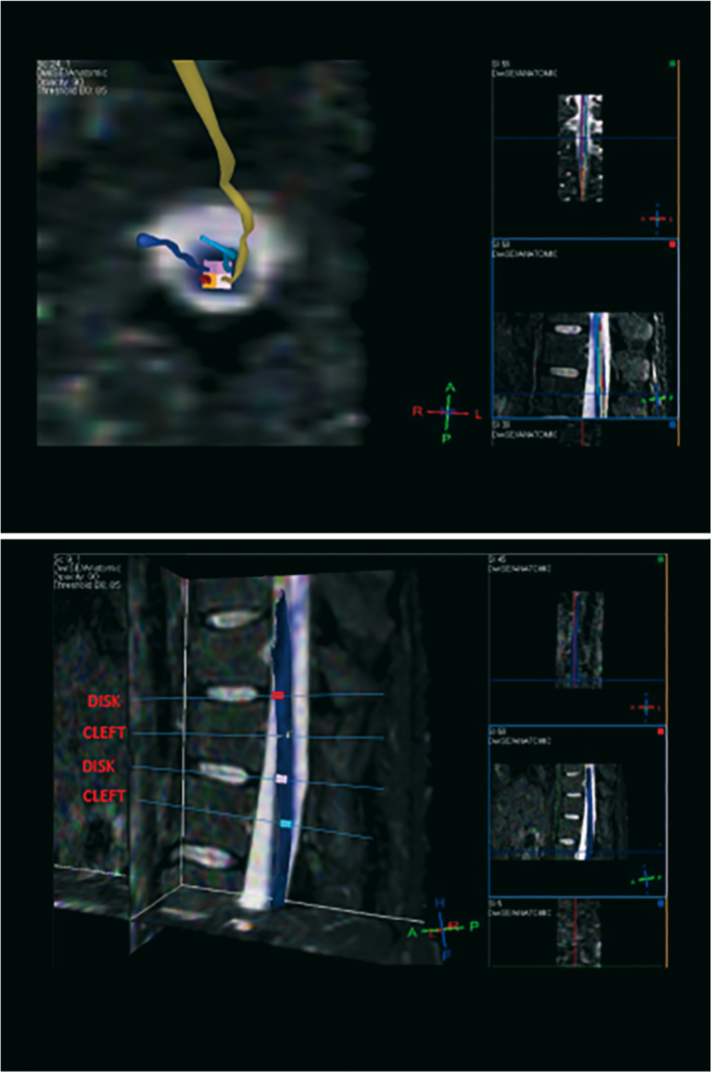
Spinal cord MRI. Upper Panel: Diffusion Tensor Imaging (DTI) overlaid with the STIR pulse sequence, in a MPR algorithm. For each of single point (colored box) ROIs, a correspondent fiber is evoked and reconstructed to ensure that the level examined and the ROI adopted is inside the spinal cord. Lower Panel: DTI post-processing by mean of a MPR algorithm, with overlaying of the STIR pulse sequence, to select the exact levels to be studied at this time of examination and in further MR follow-up scans. Disk and somatic vascular cleft are adopted to select the proper planes.
